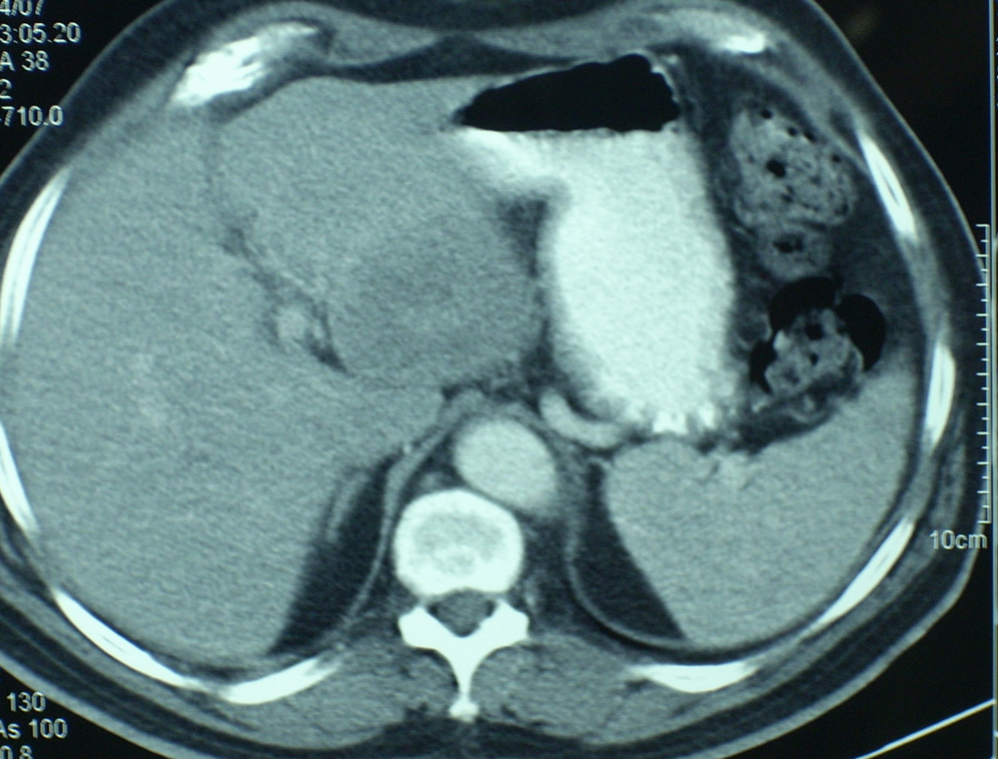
Left lobe liver metastatic lesion of size 6 cms. in thoracic-abdominal-pelvic CT performed in February 2007.
